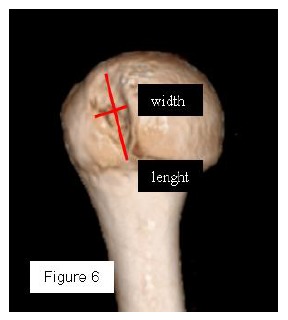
3D-CT humeral en face view with scapula elimination: the most accurate method to quantify the humeral bone defect (the minor axis is the width measure and the major axis is the lenght) 49x56mm (150 x 150 DPI).
